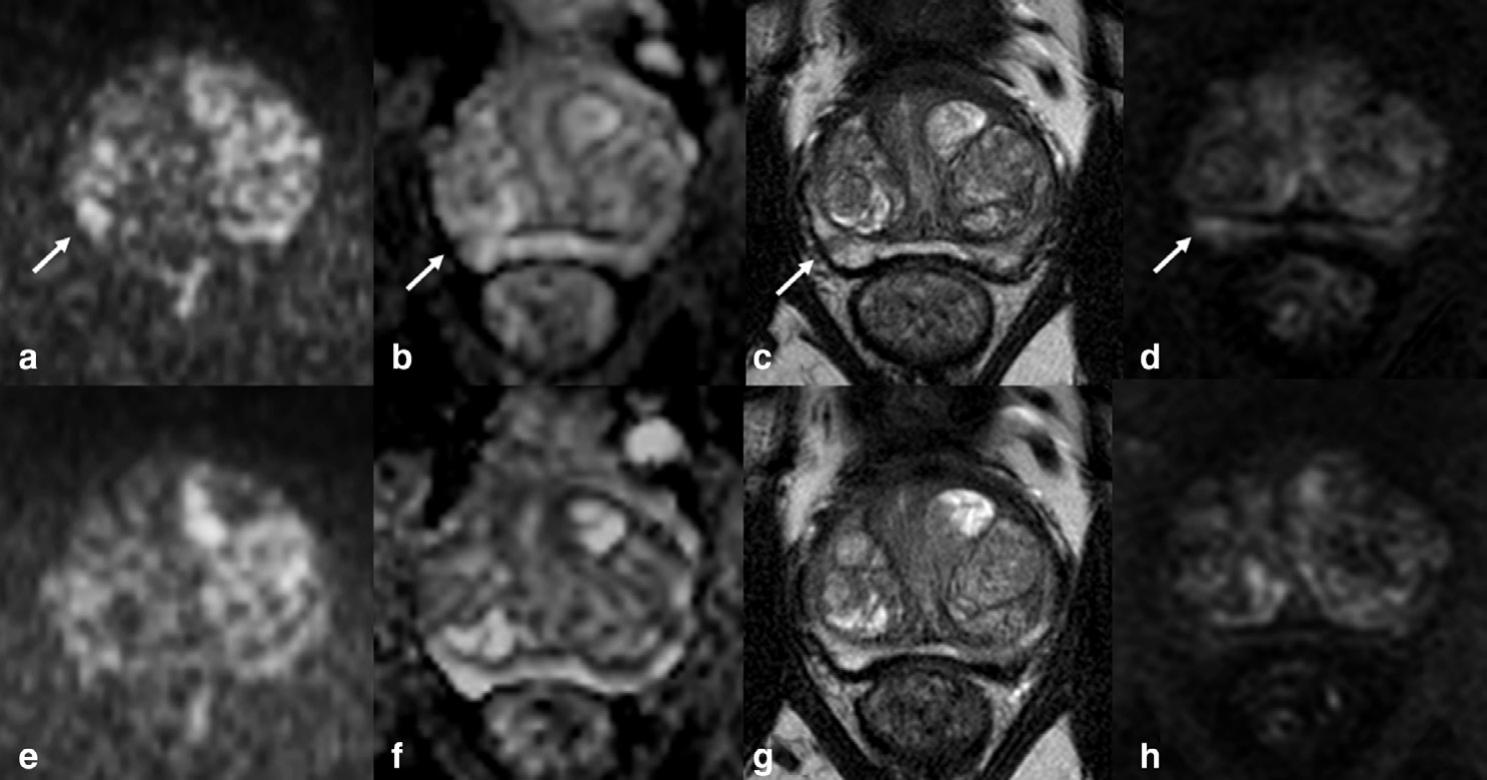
False-positive PI-RADS four index lesion in a 64-year-old patient with low PSAD (0.09) ng/ml ml^−1^ and negative-targeted and systematic biopsy. mpMRI showed a 5 mm focus of restricted diffusion in the lateral posterior peripheral zone of the right mid-gland, appearing as an hyperintense focus on high b-value image (arrow in a), and tiny focal hypointensity on the ADC map (arrow in b) corresponding to mild hypointensity on axial T2-weighted imaging (arrow in c) and focal contrast-enhancement on dynamic contrast-enhanced imaging (arrow in d). Of note, the lesion was no longer visible on the same sequences (e-h) of a subsequent mpMRI performed 6 months after the biopsy. On this basis, the lesion was retrospectively assessed as focal prostatitis.
